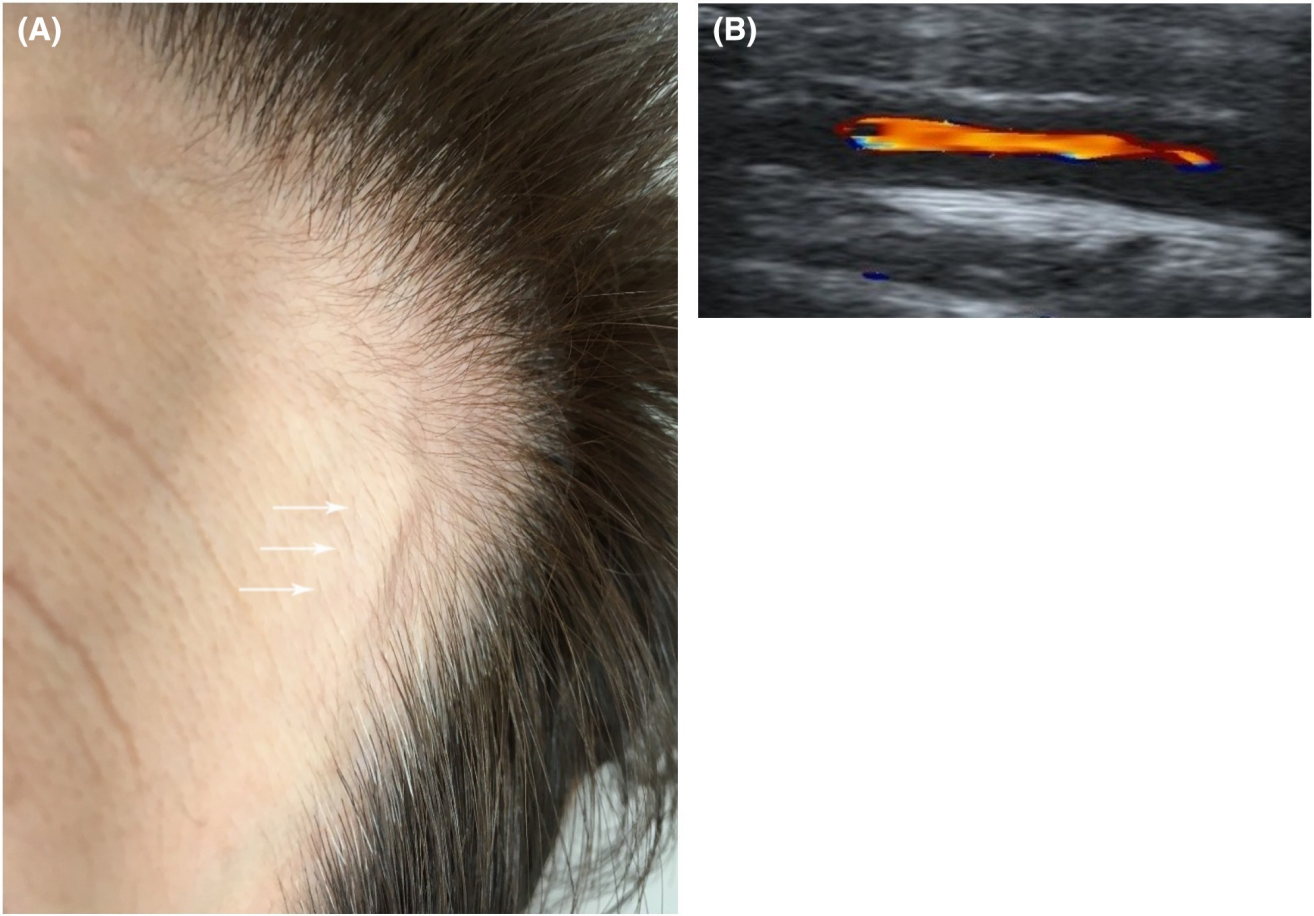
(A) Slightly enlarged left temporal artery (arrows). (B) Ultrasonography revealing wall thickening of the left temporal artery frontal branch.
